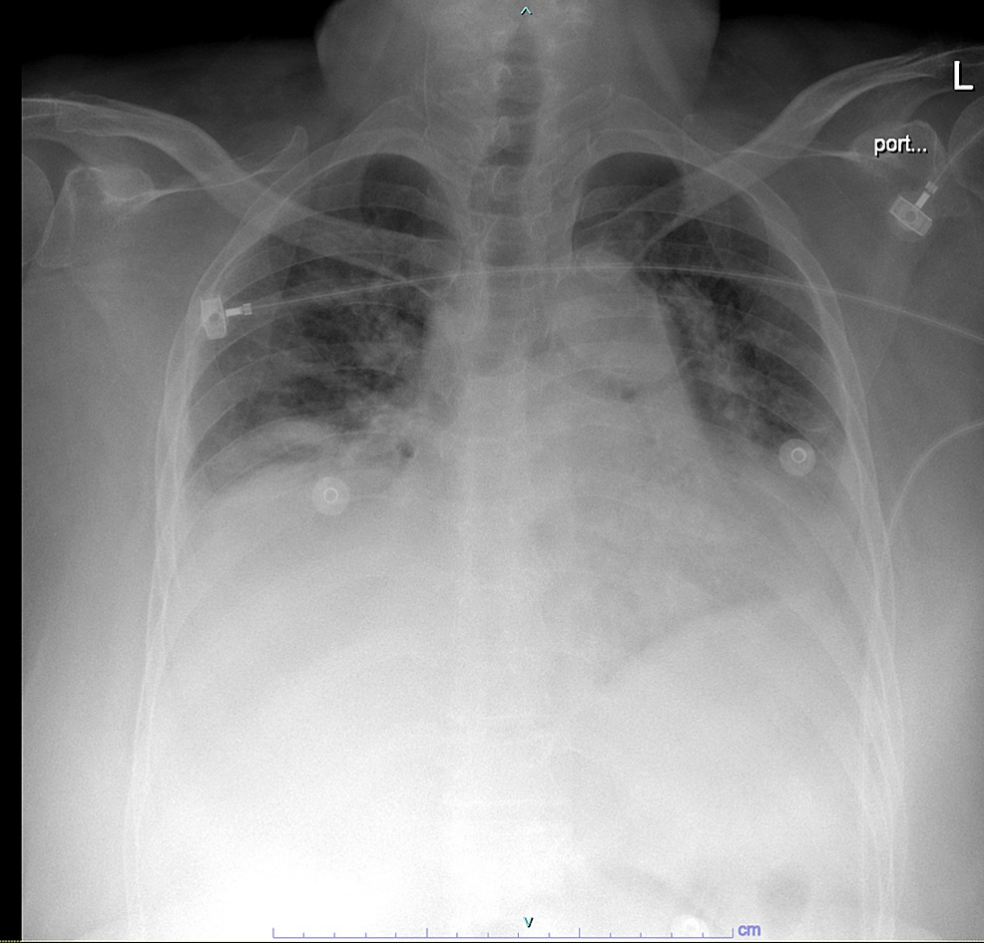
Chest X-ray shows bilateral pulmonary infiltrates and consolidation.
